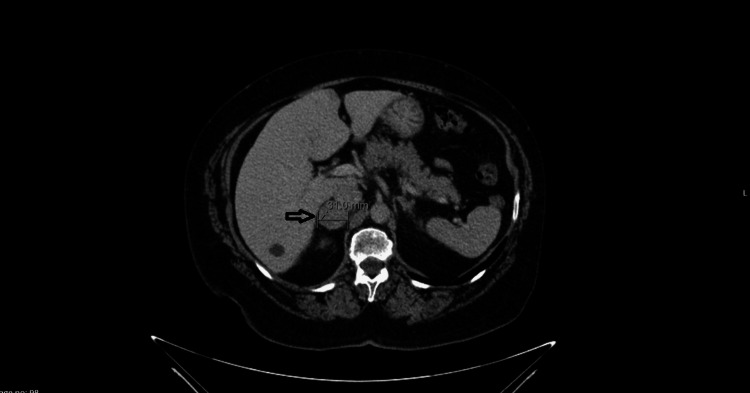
Abdominal CT scan showing a homogeneous lesion, with 31 mm of diameter, in the right adrenal gland
